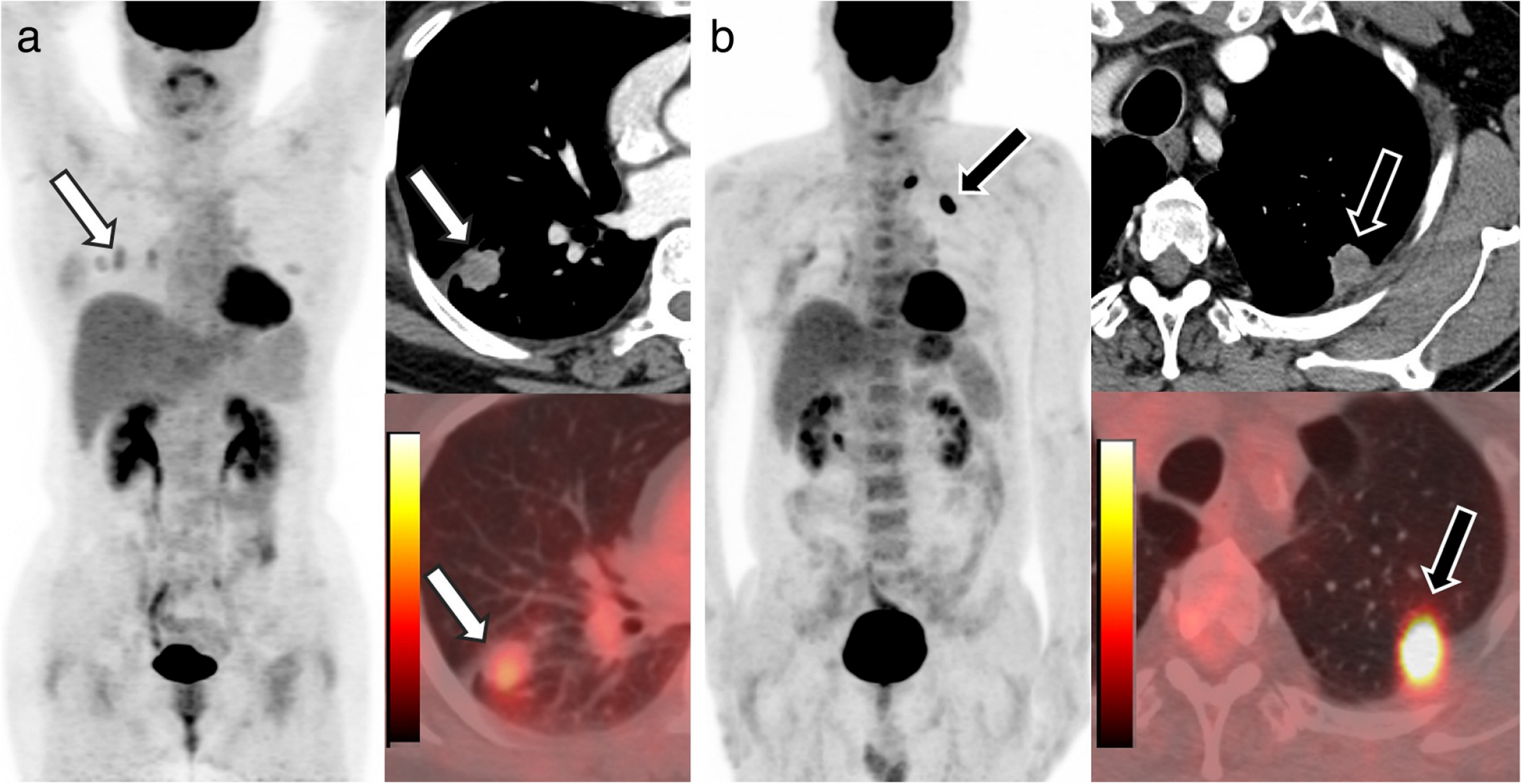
Representative figures of differences in FDG uptake according to EGFR status. a A 55 yo female with 21 mm sized adenocarcinoma in the right lower lung superior segment. SUVmax was 2.2, and TLG was 4.0. b A 58 yo female with 20 mm sized adenocarcinoma in the left upper lung apical segment. SUVmax was 14.6, and TLG was 31.5
